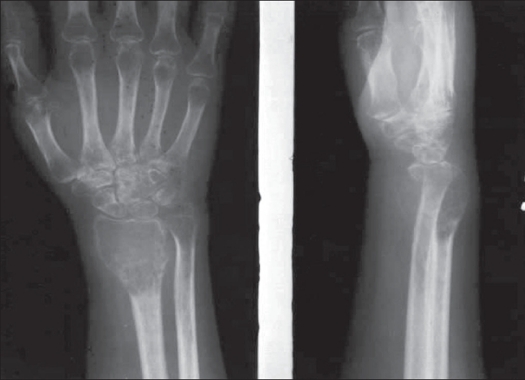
Antero-posterior and lateral x-rays of a GCT of distal radius showing cortical expansion and areas of cortical erosion
